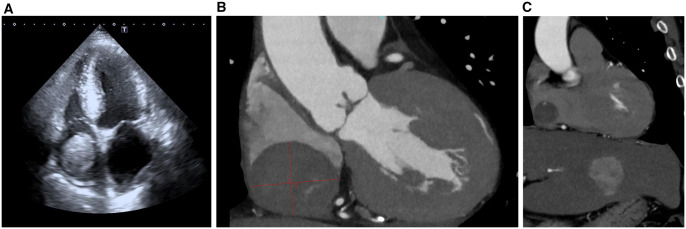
Preoperative Images. (A) Preoperative TTE, (B) Preoperative CT, (C); Liver CT.
